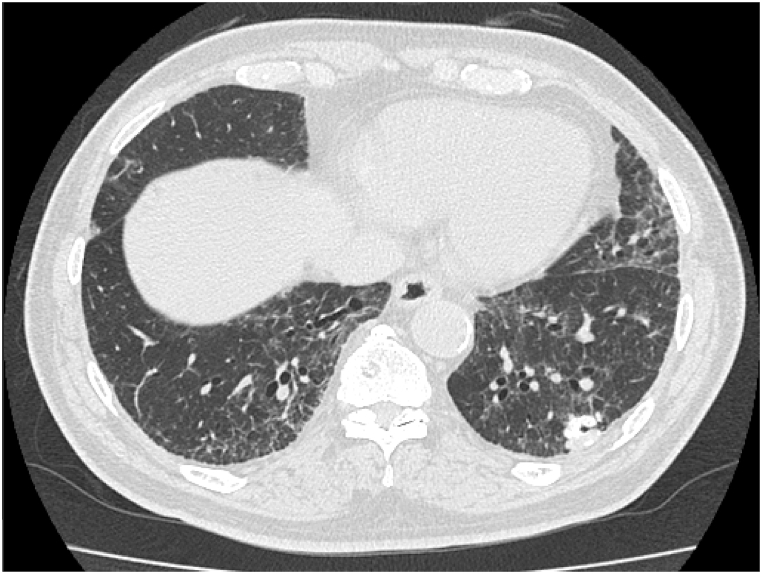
Chest computed tomography scan revealed ground-glass opacity in the basal area of both lungs and nodule in the left lower lobe.
